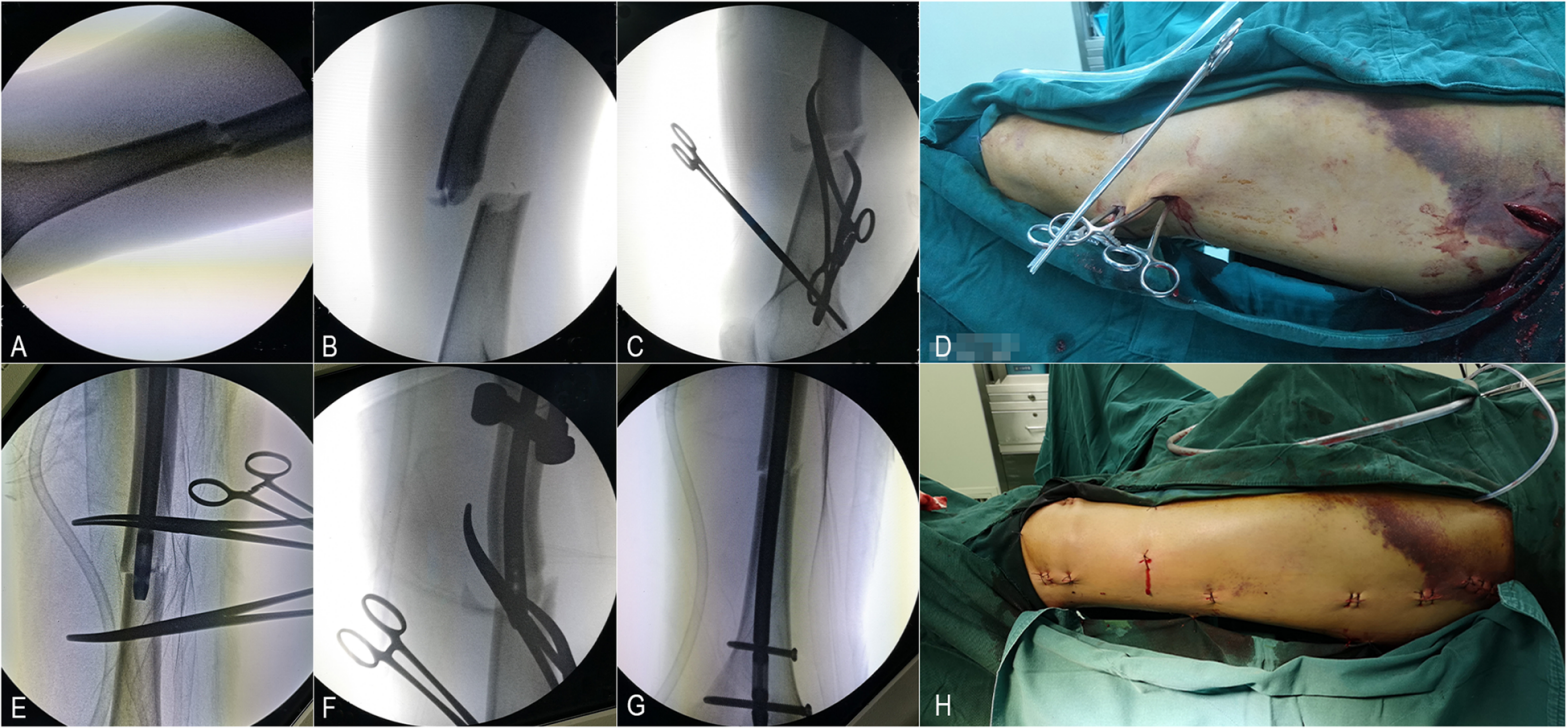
The view of the surgical procedure for this patient are shown in Fig. 2. a The “plane a” view showed that the shortening of the limb and lateral displacement of the fragment were nearly resolved after adducted traction on the fracture table. b The “plane b” view shows that the sagittal geometry was unstable and that the proximal fragment was flexed while the distal fragment sagged posteriorly. c The anterior-posterior displacement was reduced with two long, curved haemostatic forceps utilizing the lever principle. d The intraoperative photograph and e anterior-posterior and f lateral X-rays showed that the reduction was maintained by the 2 interlocking haemostatic forceps during the insertion of the IM nail. g After IM nailing. h Minimally invasive incision
